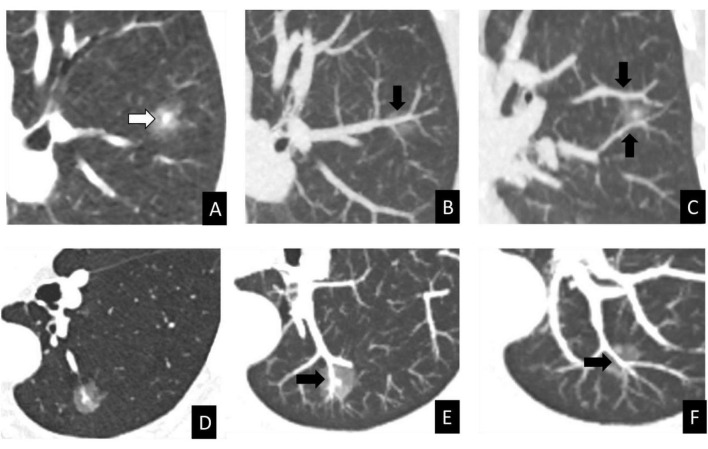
CT vascular signs in benign and malignant PSNs with similar morphological features. (A–C) Inflammatory nodule in a 68-year-old female. Axial CT images in lung window setting (A) show a PSN in the left upper lobe with irregular shape, lobulation. The MIP image (B) reveals a type II CT vascular sign (black arrow, N2 = 1). The MIP image (C) reveals a type I CT vascular sign (black arrow, N1 = 2). (D–F) Minimally invasive adenocarcinoma in a 64-year-old female. Axial CT images in lung window settings (D) show a PSN in the left lower lobe with irregular shape, lobulation. The MIP image (E,F) display type IV CT vascular signs (black arrow, N4 = 2). MIP, maximum-intensity projection; PSN, part-solid pulmonary nodules; N, number.
